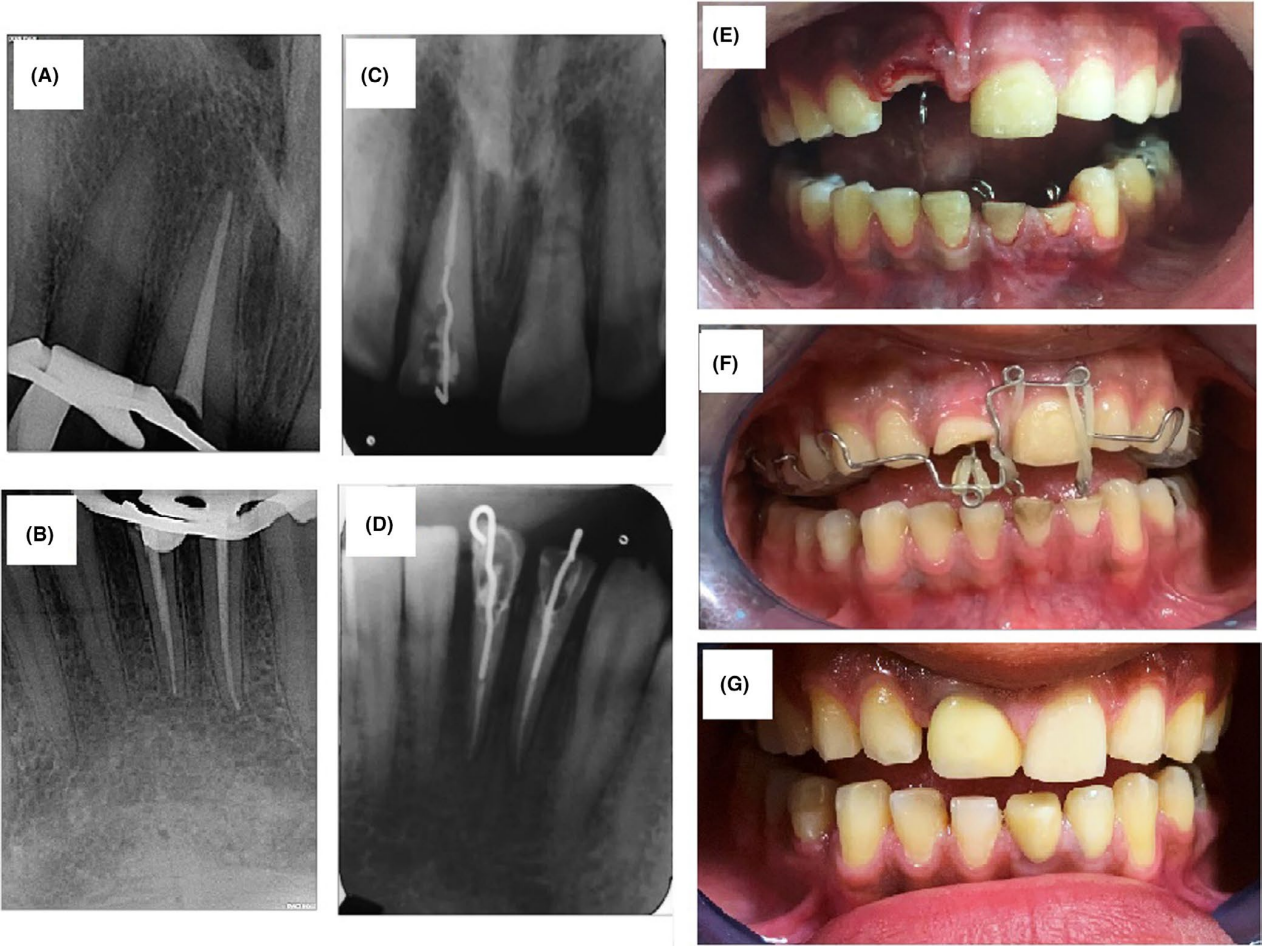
Postoperative radiograph of root canal treatment of tooth #8 (A) and teeth #23, #24 (B), Radiograph of hook cementation inside root canals of tooth #8 (C) and teeth #23, #24 (D), clinical images before (E) and after (F) application of orthodontic appliance and elastics and cemented post and core in 6‐mo follow‐up (G)
